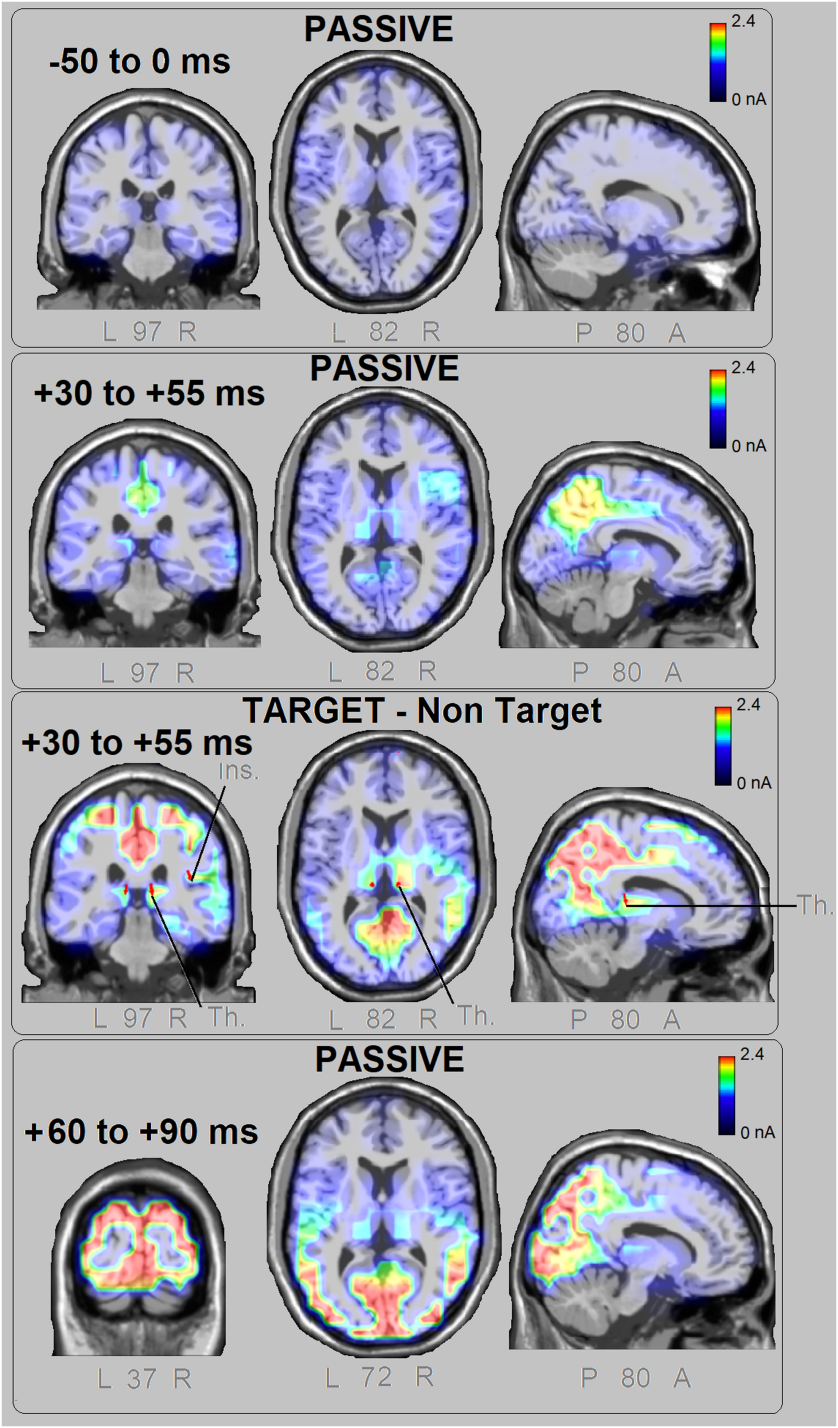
Coronal, axial and sagittal views of swLORETA source reconstruction of surface potentials performed in different pre‐stimulus and post‐stimulus attentional conditions. The various colours represent differences in the magnitude of the electromagnetic signal (nA). The electromagnetic dipoles appear as arrows and indicate the position, orientation and magnitude of the dipole modelling solution applied to the ERP waveform in the specific time window. L, left; R, right; P, posterior; A, anterior; numbers refer to the displayed brain slice in the MRI imaging plane
